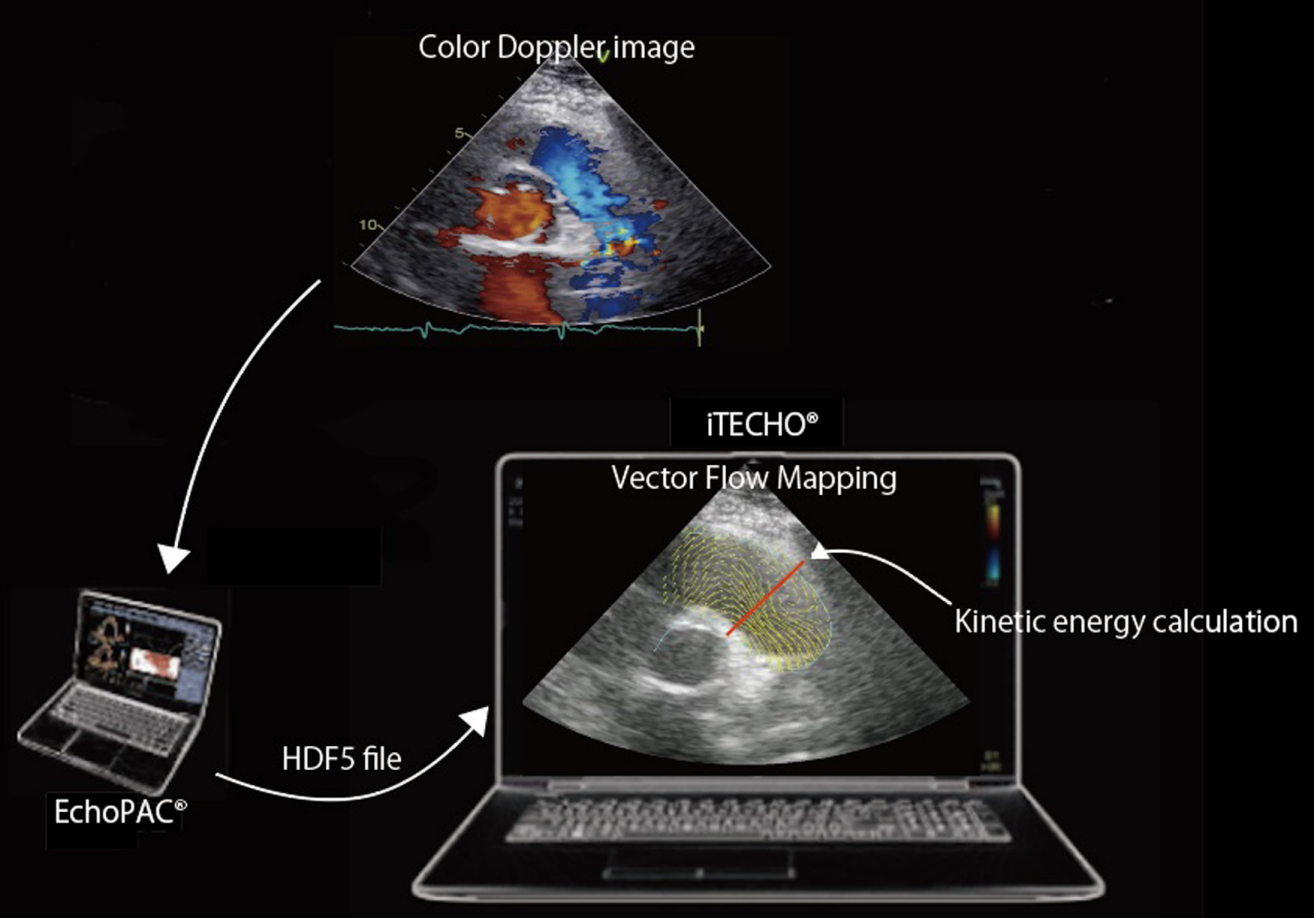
Color Doppler cine-loop image of the parasternal RV outflow view and its corresponding Vector Flow Mapping image. The stored cine-loop image is transferred to EchoPAC® (GE Healthcare, Chicago, USA) and converted into HDF-5 files. The HDF-5 file is imported into the VFM software (iTECHO®) and analyzed. Kinetic energy can be calculated from the vectors passing through RVOT (red line) over one cardiac cycle.
